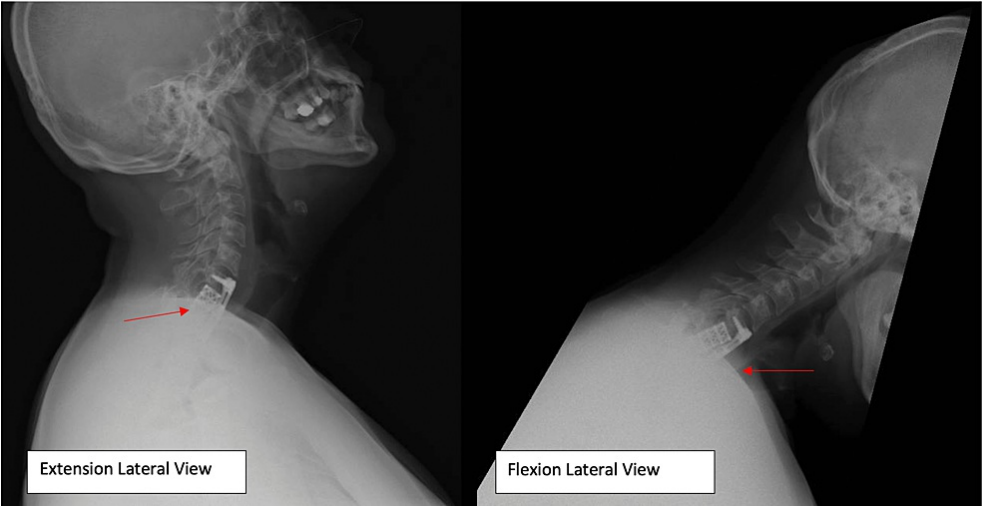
Latest lateral X-rays at the last follow up; flexion and extension views
